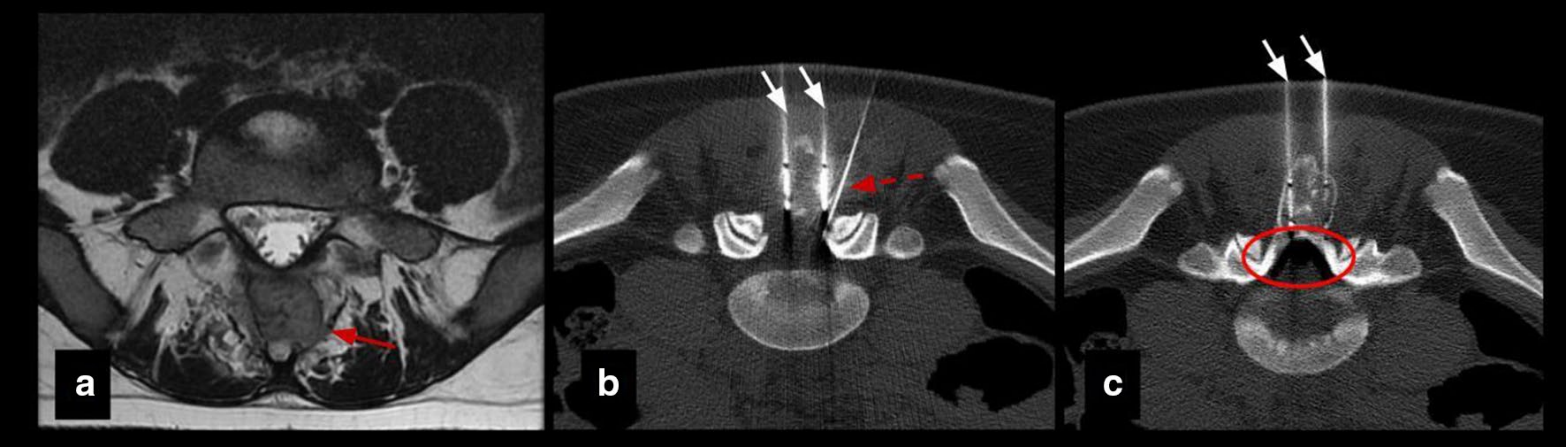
Insulation technique: epidural pneumo-dissection. Osteoblastoma in a 17-y.o patient. a Axial T2 MR image shows an expansile lesion in the posterior elements of L5 (red arrow). b, c Axial CT images show cryoprobes (white arrows) and epidural needle (dashed arrow) for pneumo-dissection system (circle) used to protect L4 and L5 nerve roots
